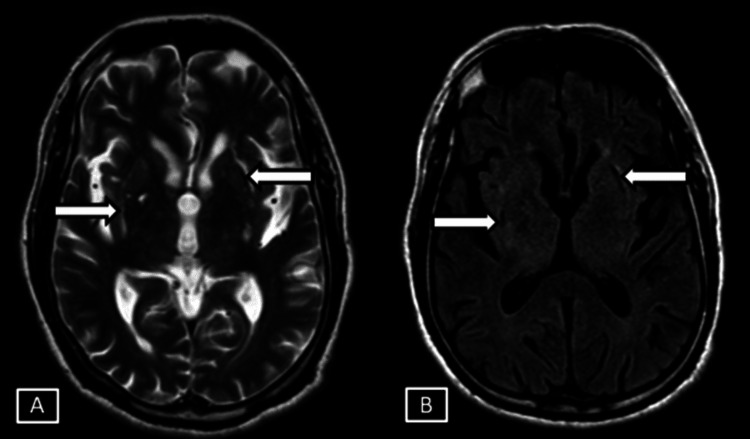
Case 3 MRI brain T2 (A) and FLAIR (B) sequences. Images show chronic infarct changes with surrounding gliosis seen involving the right lentiform nucleus and left external capsule represented by the white arrows. FLAIR: Fluid Attenuation Inversion Recovery
